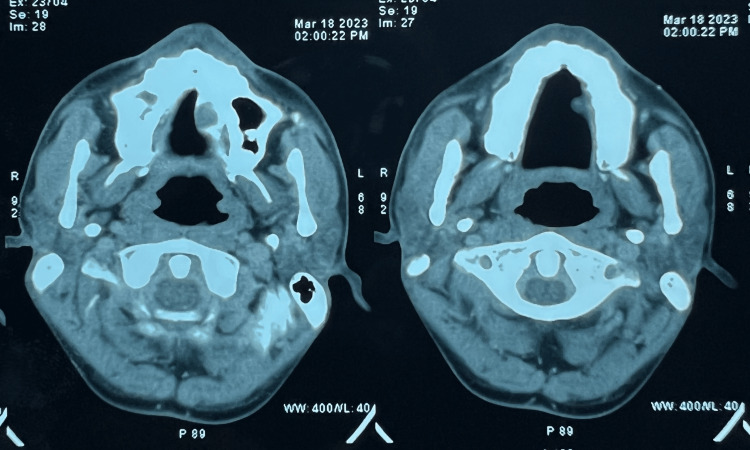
CT scan image showing the soft tissue lesion
